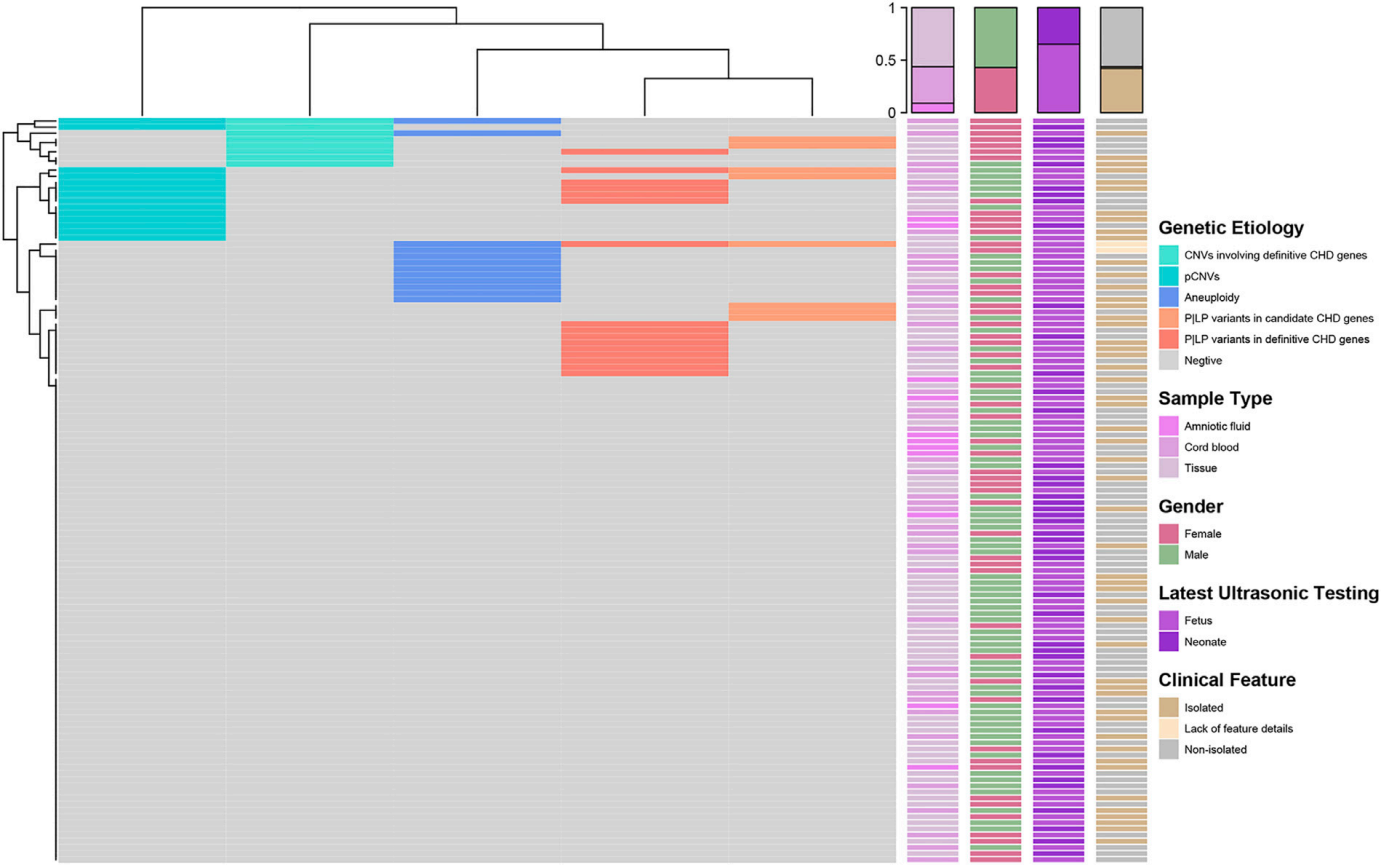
Landscape of distribution of genetic etiology and clinical features (*N* = 121). The heatmap on the left demonstrated the distribution of genetic etiologies detected in each sample. Each row represents a sample and the columns are five categories of genetic etiology. The correspondence colors of genetic etiology were summarized in the right legend. Four clinical characteristics including sample types, gender, latest ultrasonic testing, and clinical features of each sample were demonstrated in the right heatmaps separately. At the same time, we also displayed the distribution statistics of clinical characteristics on the top of the heatmap.
